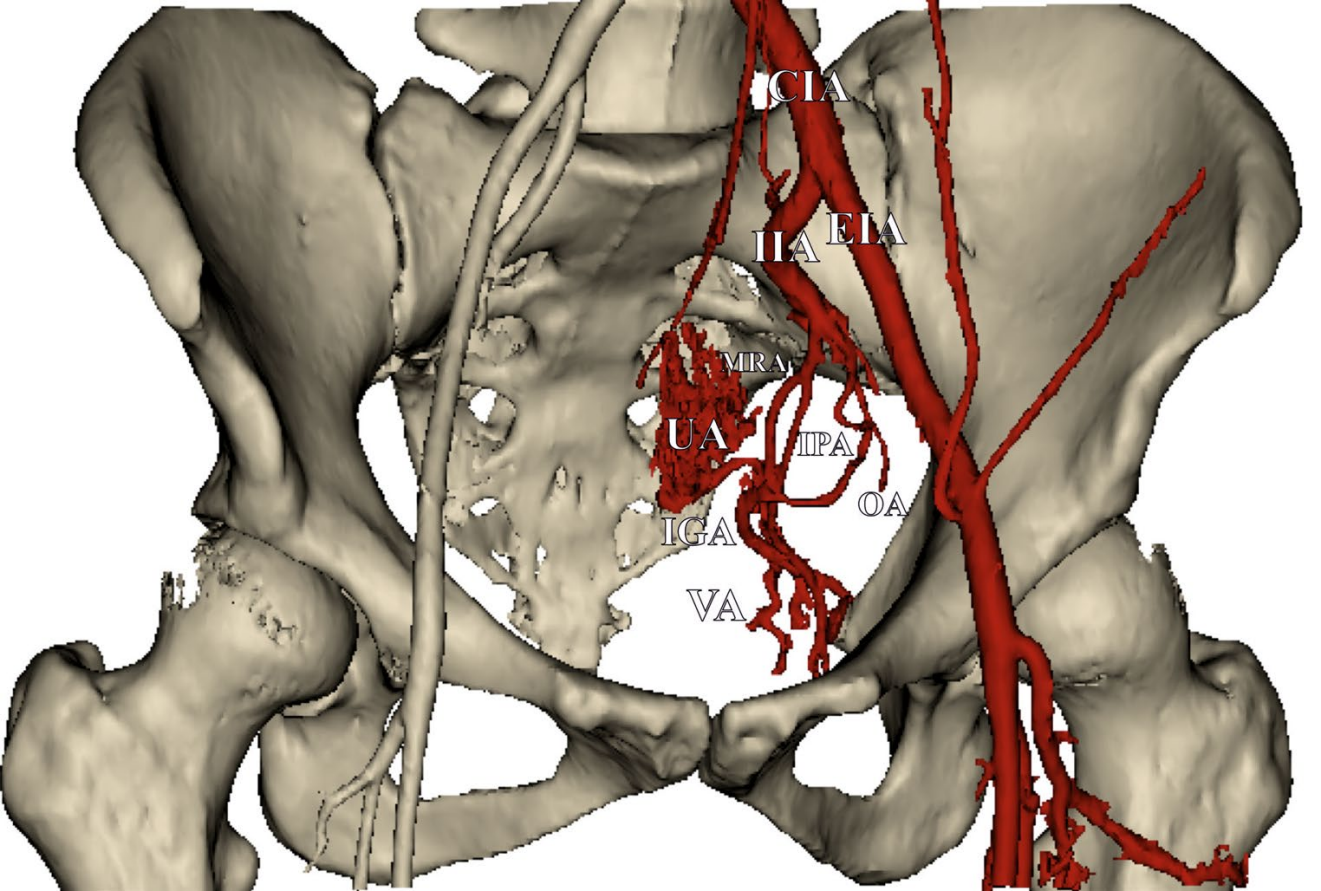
A three-dimensional, computed tomography angiography of the studied area. Some of the arteries were removed in order to provide beter visibility. UA - Uterine Artery. CIA - Common Iliac Artery. EIA - External Iliac Artery. IIA - Internal Iliac Artery. IGA - Inferior Gluteal Artery. IPA - Internal Pudendal Artery. OA - Obturator Artery. VA - Vaginal Artery. MRA - Middle Rectal Artery
